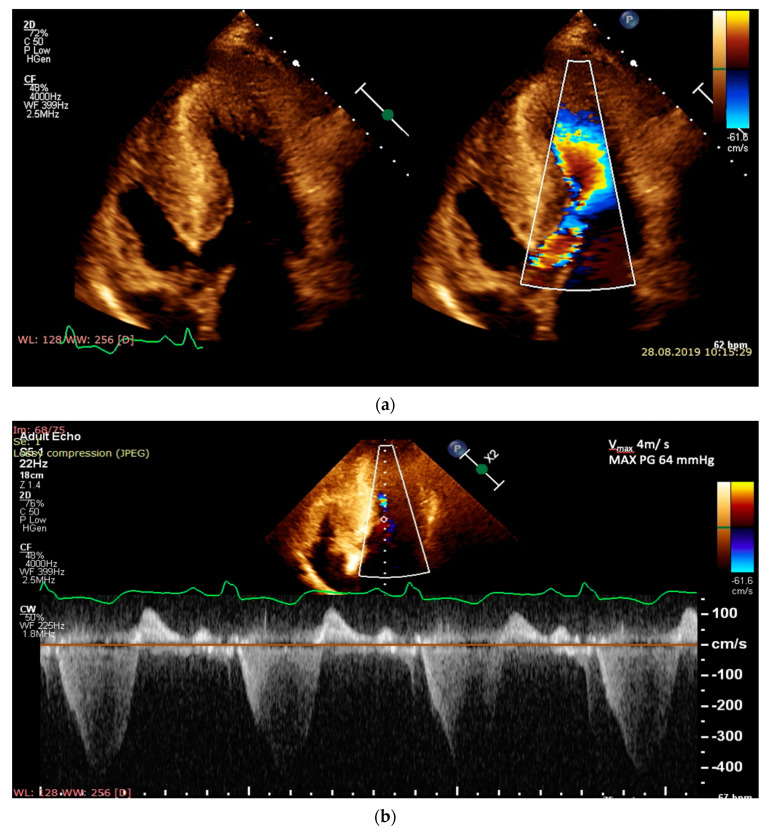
Echocardiography assessment at rest, in a 20-year-old male with obstructive hypertrophic cardiomyopathy: (a) apical five-chamber view with and without color Doppler demonstrating left ventricular mid-cavity and outflow tract obstruction, with turbulent flow; (b) continuous wave Doppler through the left ventricular outflow tract demonstrating the obstruction, with a peak gradient of 64 mmHg.
